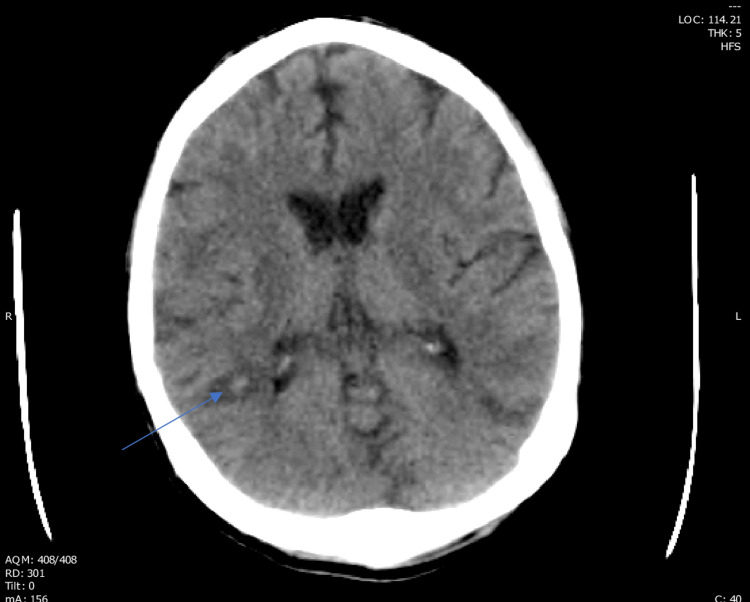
Arrow showing suspected cerebral hemorrhage surrounding the 6 mm tumor on CT head.
